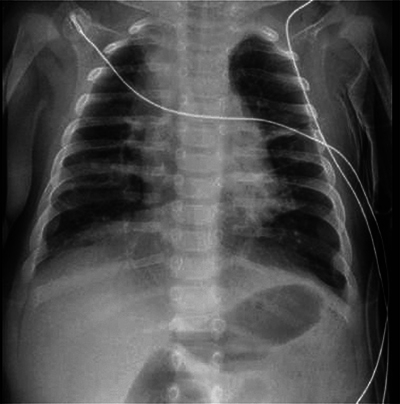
Chest antero-posterior radiograph. Multiple pulmonary opacities and an air bronchogram suggesting pneumonia.
